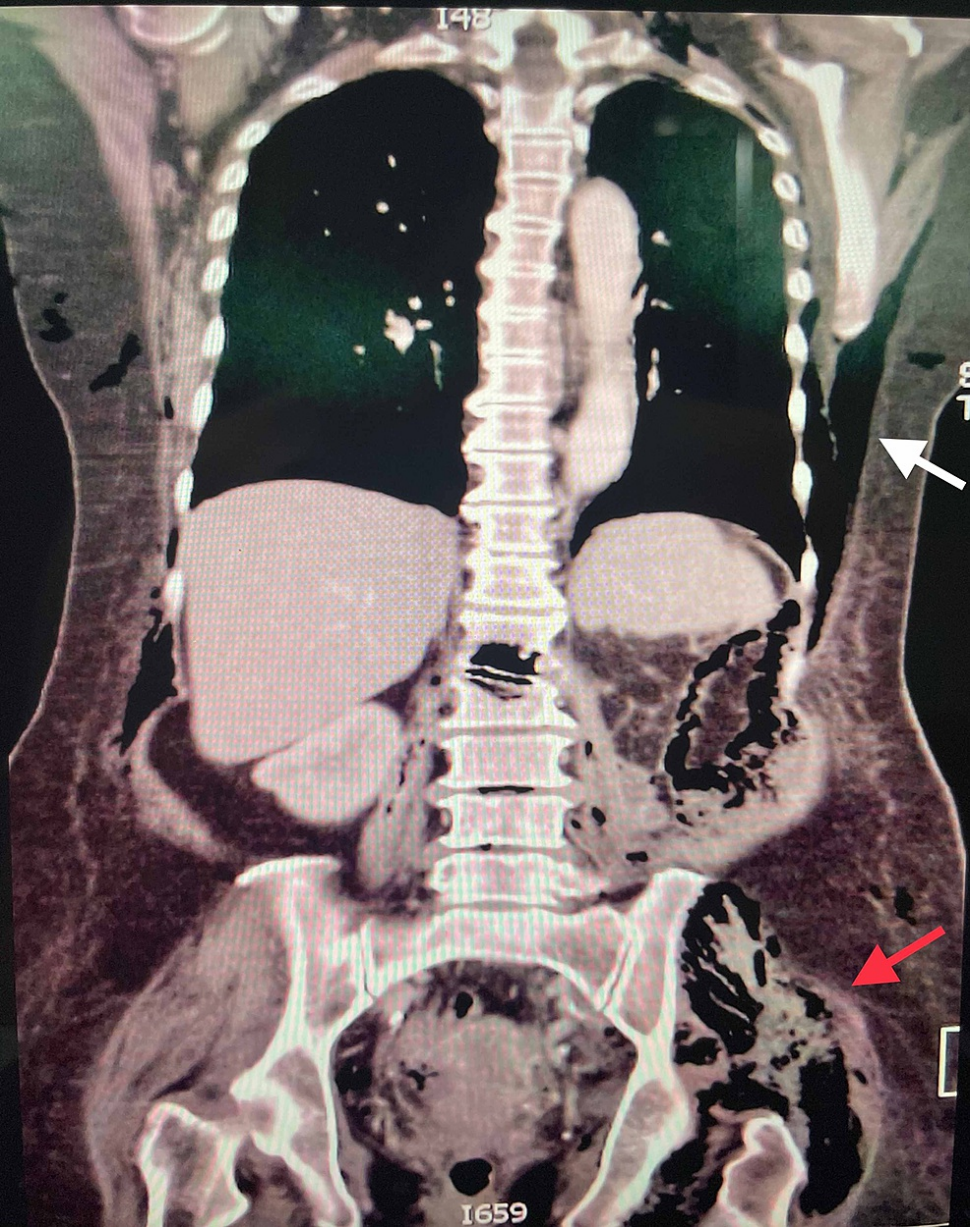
Computed tomography scan showing an abscess of the left psoas muscle (red arrow) with extensive subcutaneous emphysema (white arrow).
